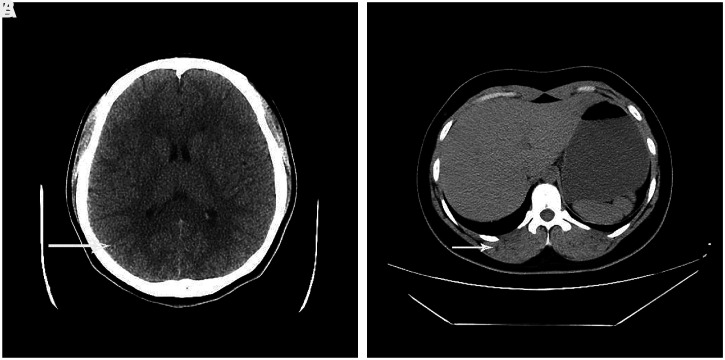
(A and B) Computed tomography scans of the head and abdomen (November 2017) reveal multiple, small low-density lesions in brain parenchyma and muscle.
